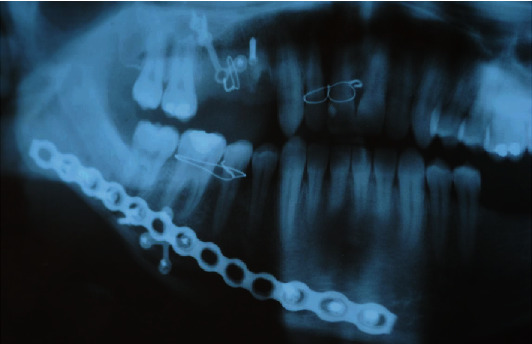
Orthopantomographic radiographic image of titanium plates and screws in position.
